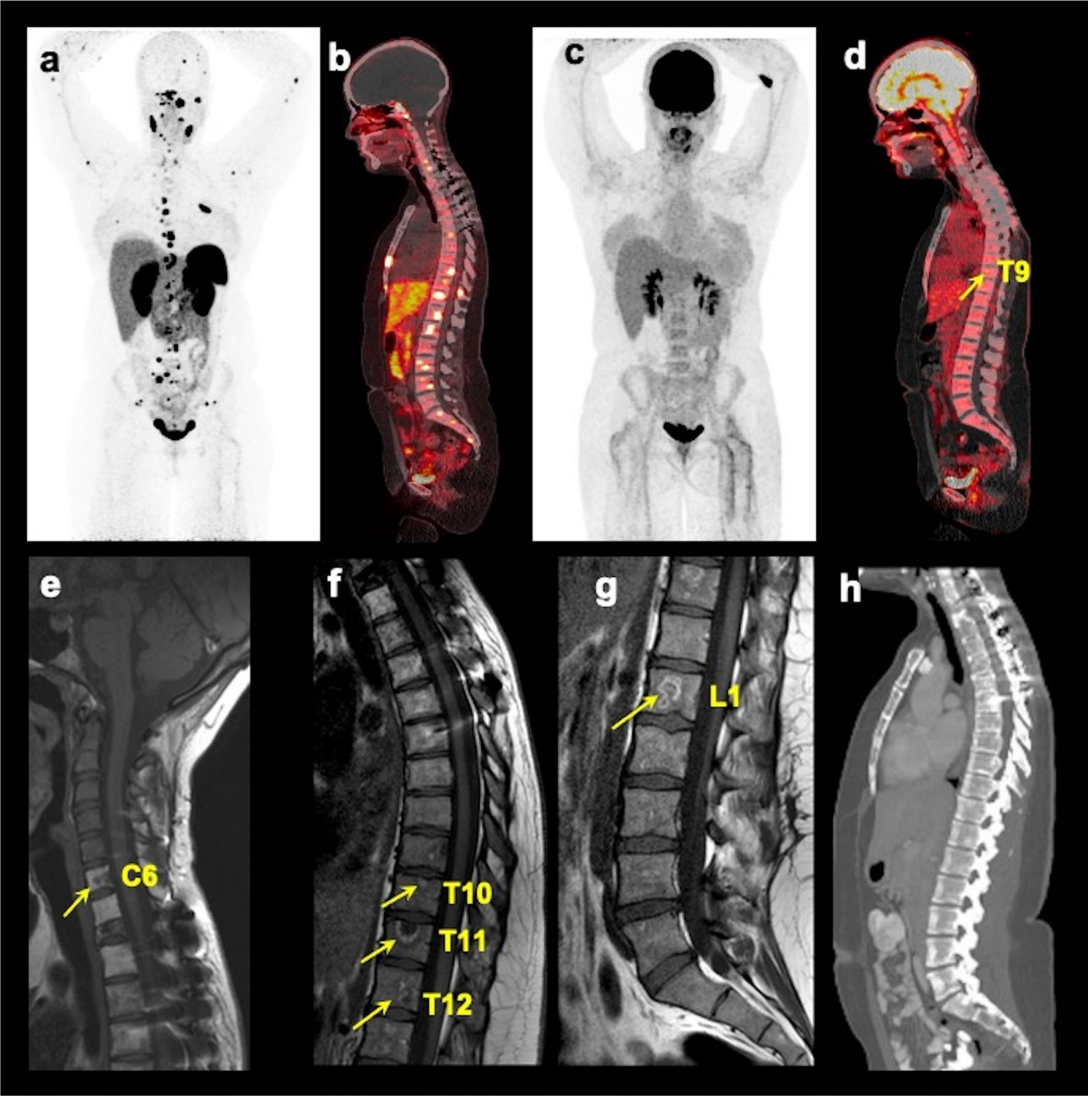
Multimodality imaging of spinal bone metastases in a pheochromocytoma/paraganglioma participant. The images of whole-body ^68^Ga-DOTA(0)-Tyr(3)-octreotate ([^68^Ga]DOTATATE; a anterior maximum intensity projection (MIP) and (b) fused sagittal PET/CT), ^18^F-fluoro-2-deoxy-d-glucose ([^18^F]FDG; c MIP and (d) fused sagittal PET/CT), T1-weighted sagittal MRI of the cervical (e), thoracic (f), and lumbar (g) spine, and contrast-enhanced CT (h sagittal) of a 25-year-old woman with germline pathogenic variant in a gene encoding for succinate dehydrogenase B subunit are shown. This figure shows superiority of [^68^Ga]DOTATATE PET/CT in the detection of additional spinal bone metastases at C2, C5, C7, T1-2, T4-8, L2, and L5 compared to [^18^F]FDG PET/CT in detecting spinal bone metastases at T9 (arrow on fused sagittal image and not appreciated on MIP image) and L4 (not appreciated on MIP and fused sagittal images), and MRI spine at C6, T10-12, and L1 (arrows), respectively. The whole-body CT was read negative for any spinal bone metastases. This participant did not undergo whole-body MRI
